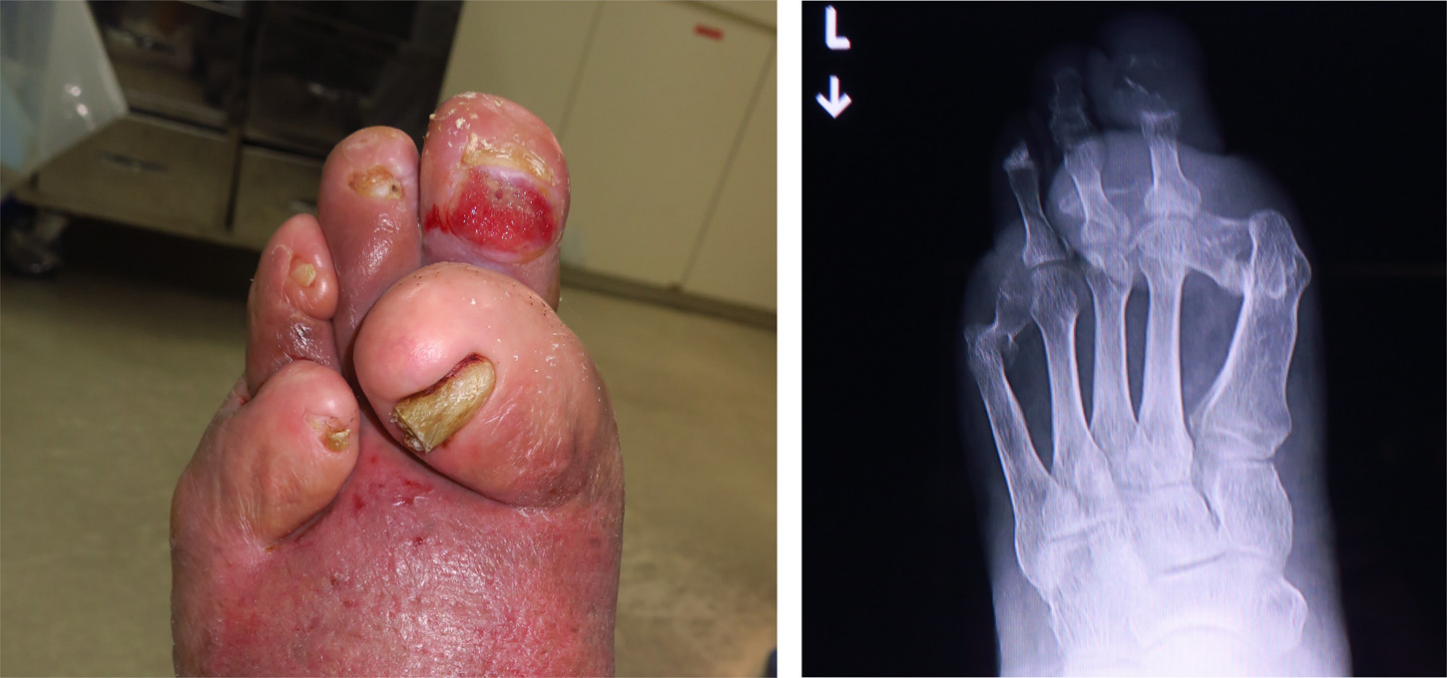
The left diabetic foot ulcer for the first time. (Left) A simple weight-bearing radiographic image of the left foot. (Right).
